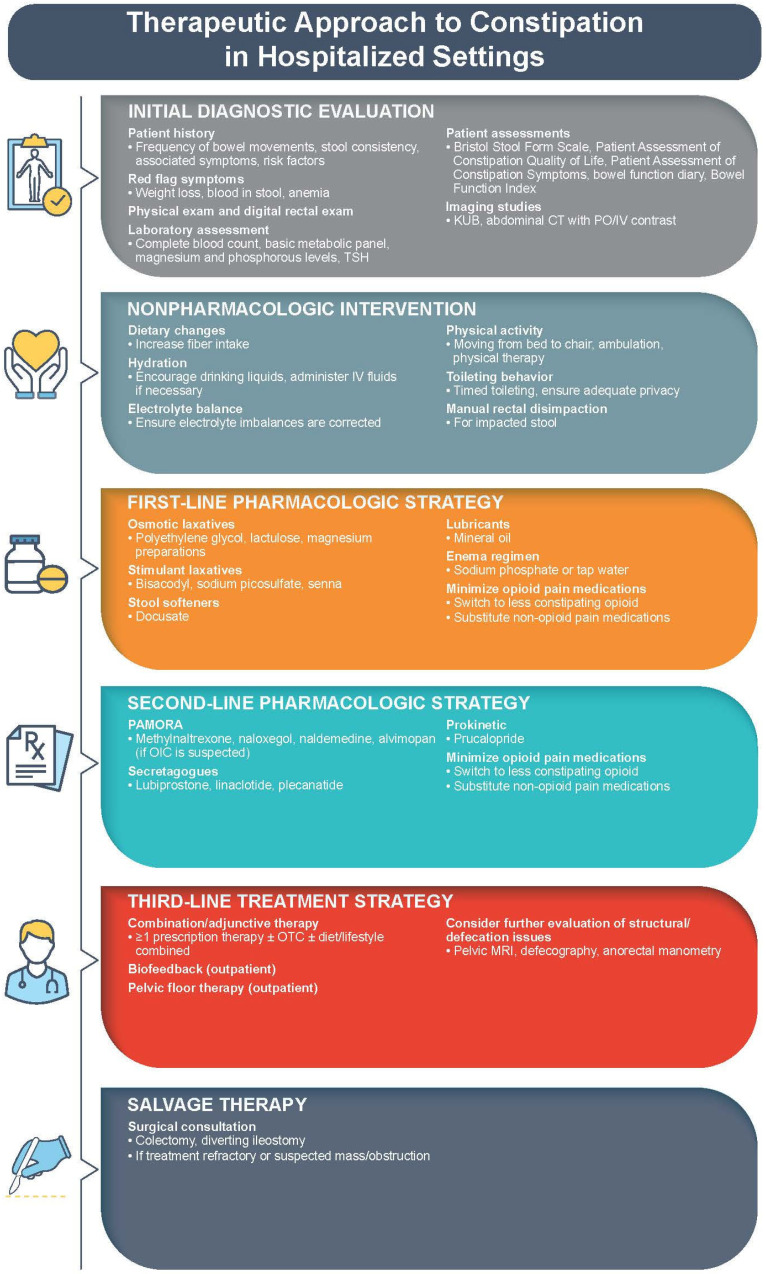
Therapeutic approach to constipation in hospitalized settings [2,7,11,16,23,29,30,31,32,33,34,35,36]. CT: computed tomography; IV: intravenous; KUB: kidney, ureter, and bladder X-ray; MRI: magnetic resonance imaging; OIC: opioid-induced constipation; PO: positive oral; TSH: thyroid-stimulating hormone.
